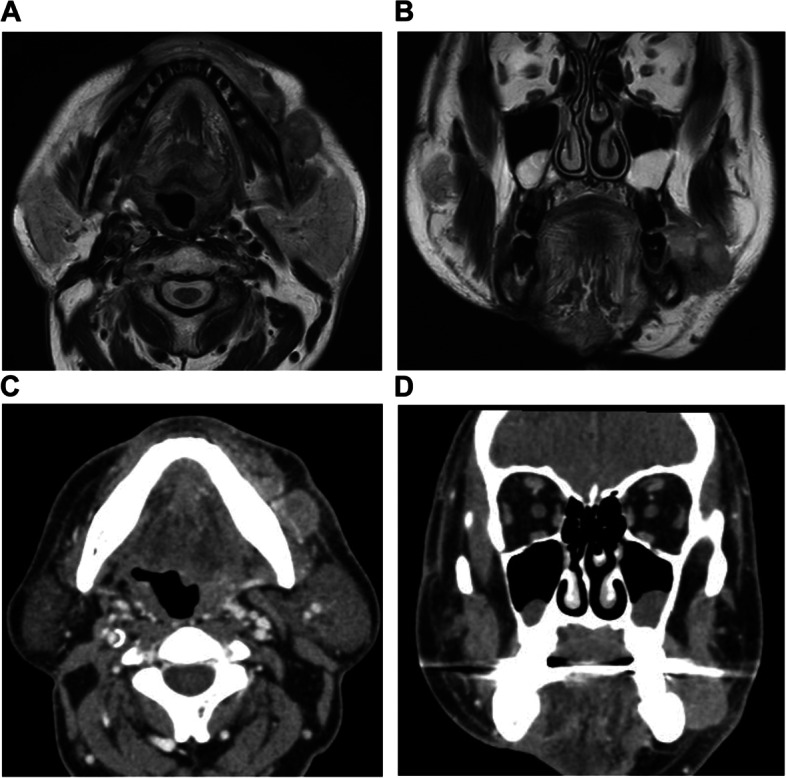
Axial (A) and coronal (B) T1-weighted magnetic resonance images and axial (C) and coronal (D) computed tomographic images, all of the head, depicted the lesion extending through the buccinator muscle toward the platysma muscle and involving the surface of the buccal skin
